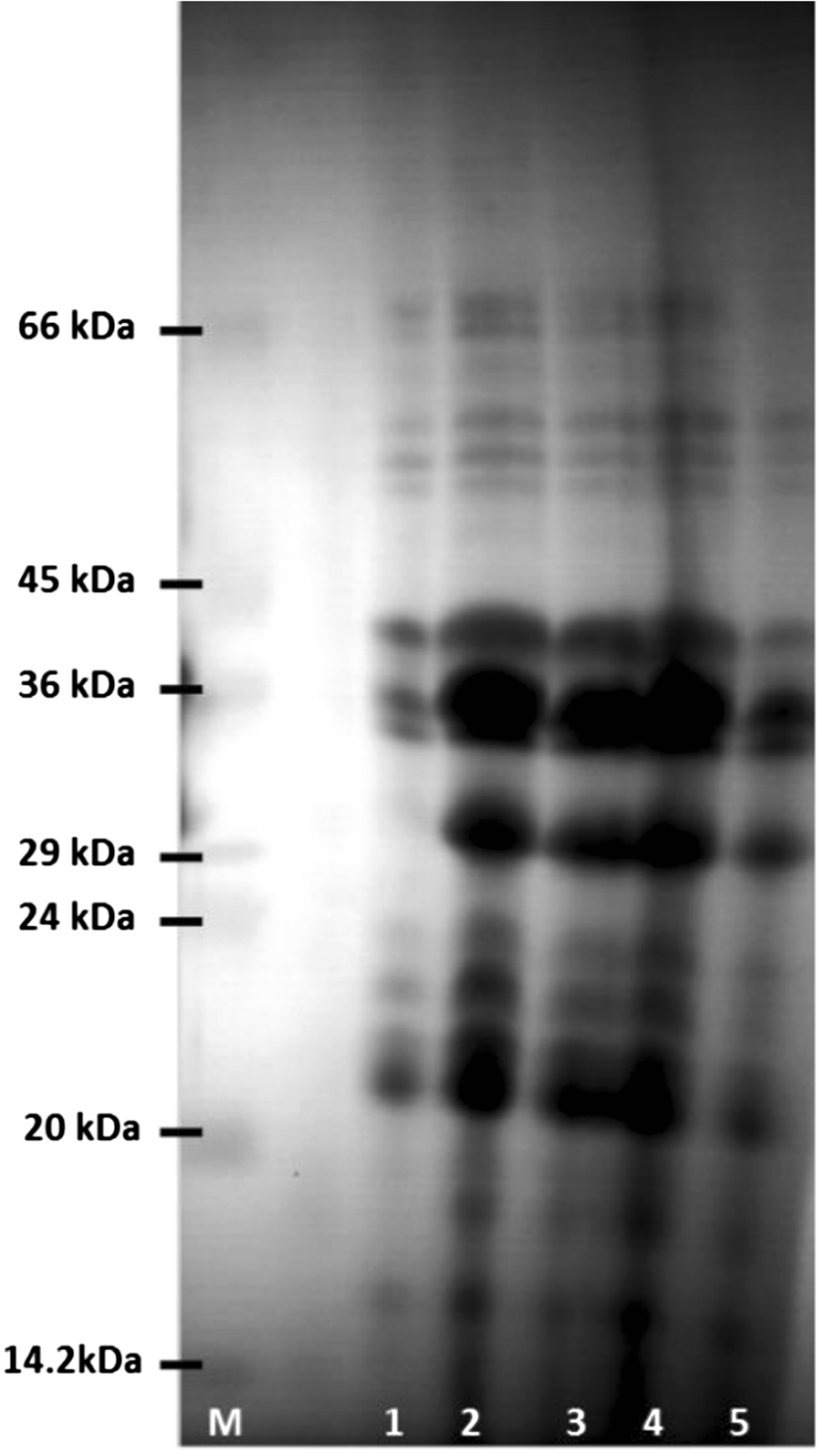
SDS-PAGE patterns of the glutelin fractions of the chickpea samples extracted with the glutenin extraction procedure. M: Marker, 1: CF, 2:CT day 0, 3: CT day 1, 4: CT day 2, 5: CT day 3.
